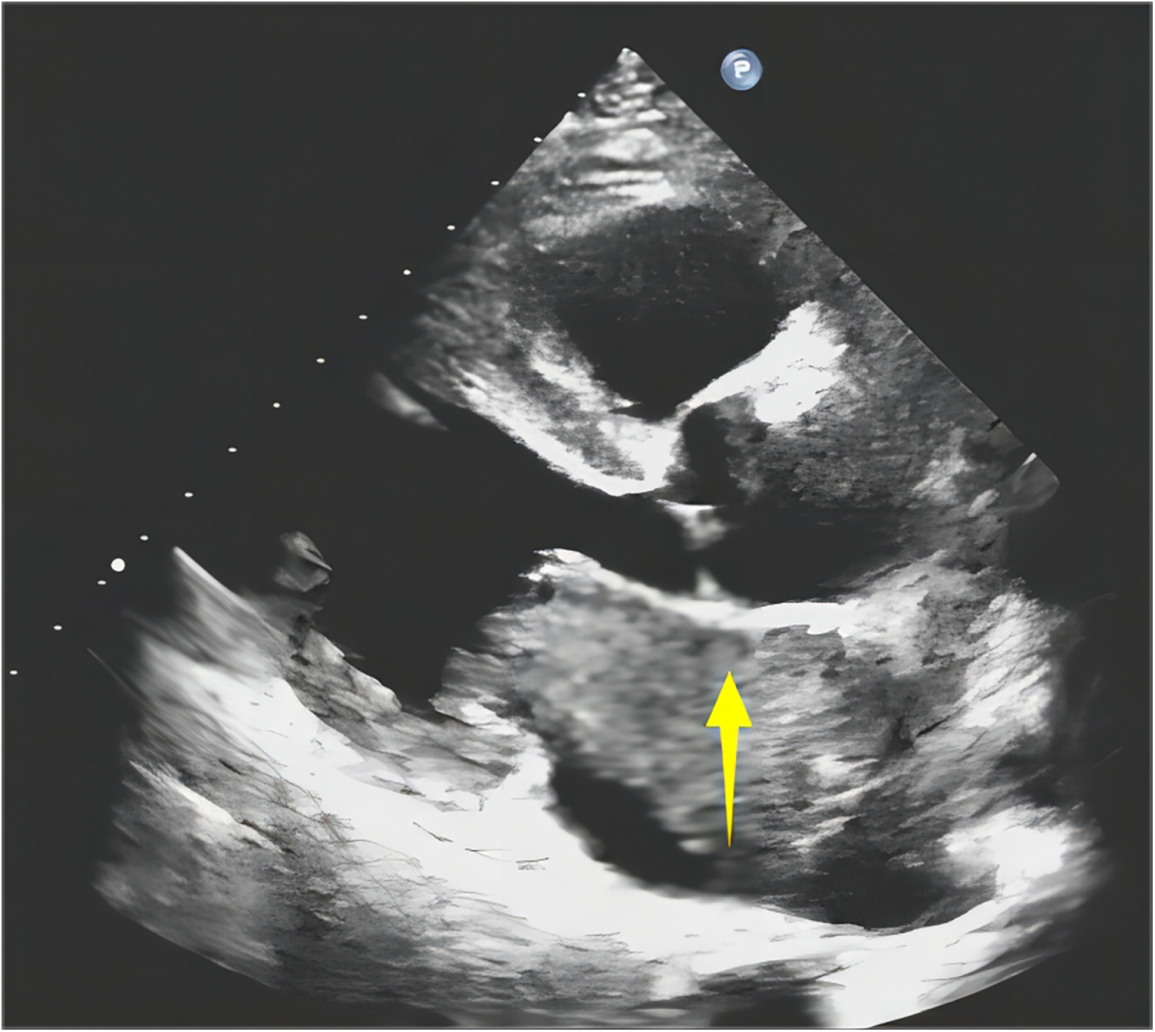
Ultrasonography shows a left atrial isoechoic mass (↑).
